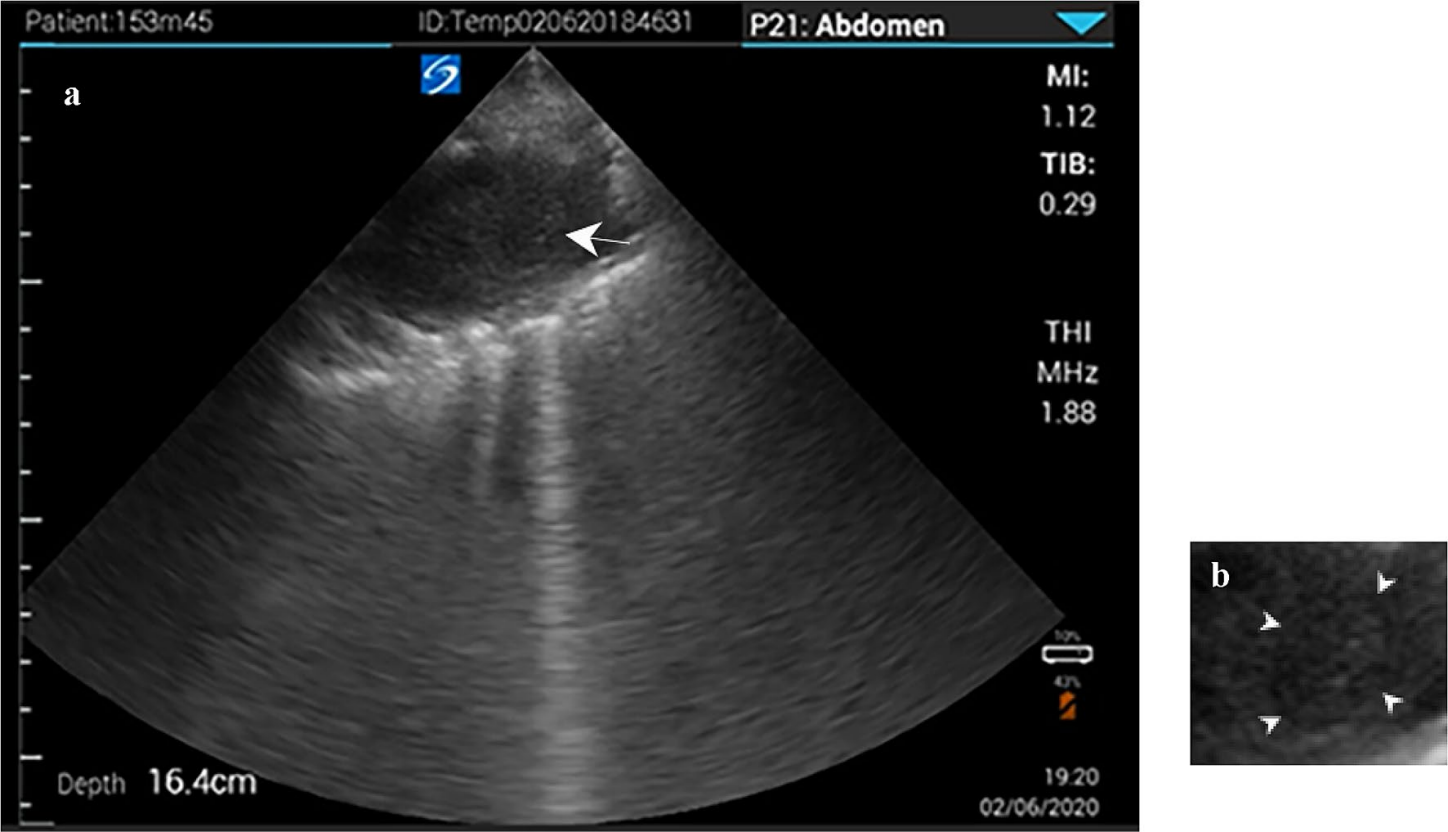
a Sonographic image with phased array probe shows oval cavity with irregular border and hypoechoic contents, the arrow marks a roundish structure with an anechoic rim. b Roundish structure, the arrowheads mark the anechoic rim
